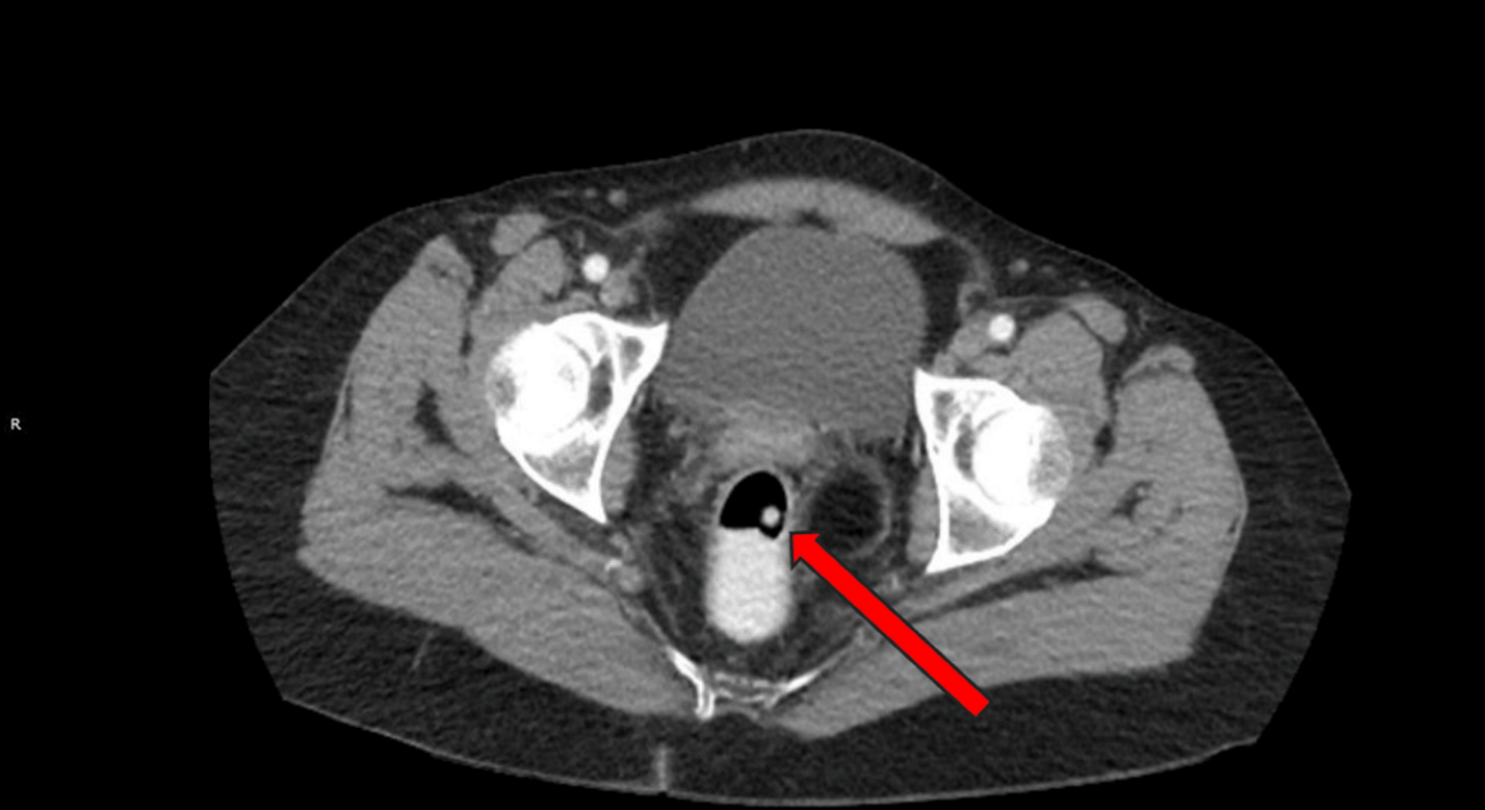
CT image of the pelvis demonstrating abnormal intraluminal air within the vaginal canal (indicated by red arrow).
